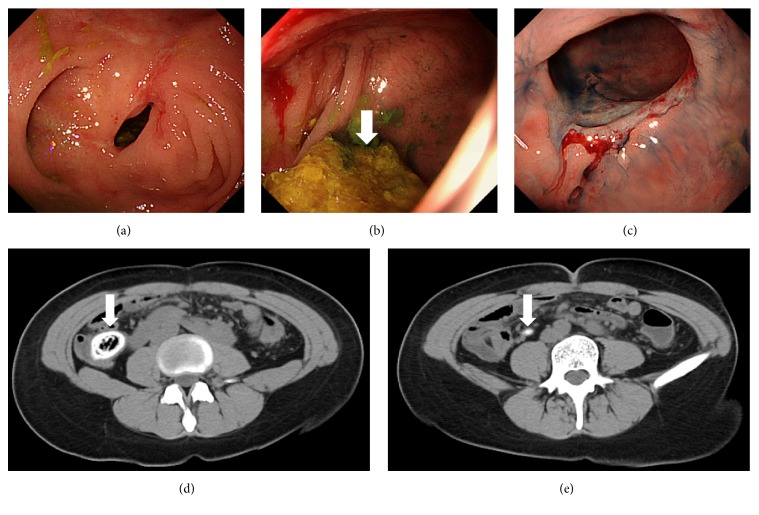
Endoscopic and radiologic images. Colonoscopy performed 15 months previously shows ulceration and stenosis (a) and a mass trapped in the semi-closed lumen in the cecum ((b), arrow). Another ulceration is observed in the anastomotic site (c). CT shows a calcified mass in the cecum ((d), arrow) and fecalomas within the appendix ((e), arrow).
